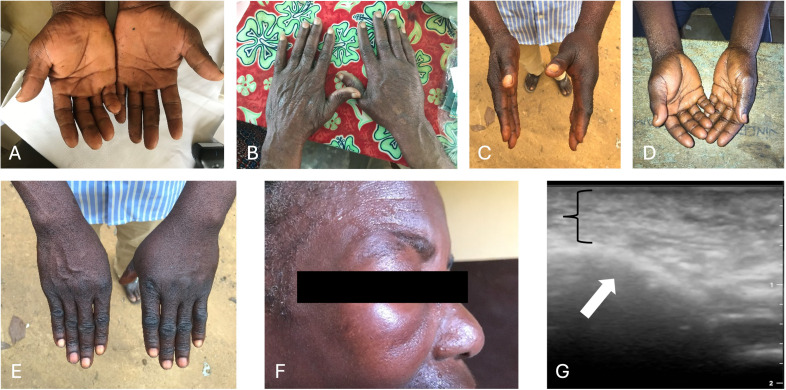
“Typical” Calabar swellings seen during the study of hands (A-E) and face (F) as well as an ultrasound image (G: white arrow indicating the bone, black bracket indicating the edema) of the orbital swelling depicted in (F). Pictures A to F were taken by LV.
